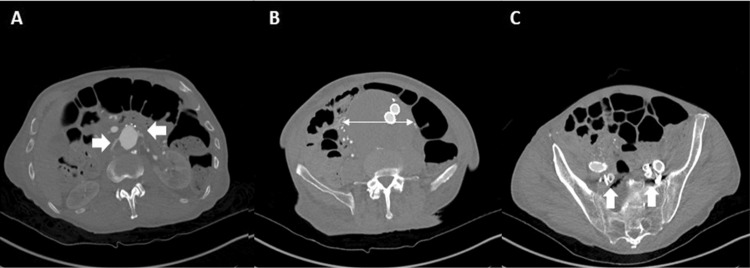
CTA of the patient on presentation A: Axial cut of CTA at the level of the right and left renal arteries (white arrows); B: Axial cut of CTA at the level of aneurysm sac's largest dimension (double white arrow); C: Axial cut of CTA at the level bilateral occluded internal iliac arteries (white arrows) CTA: Computed tomography angiography
